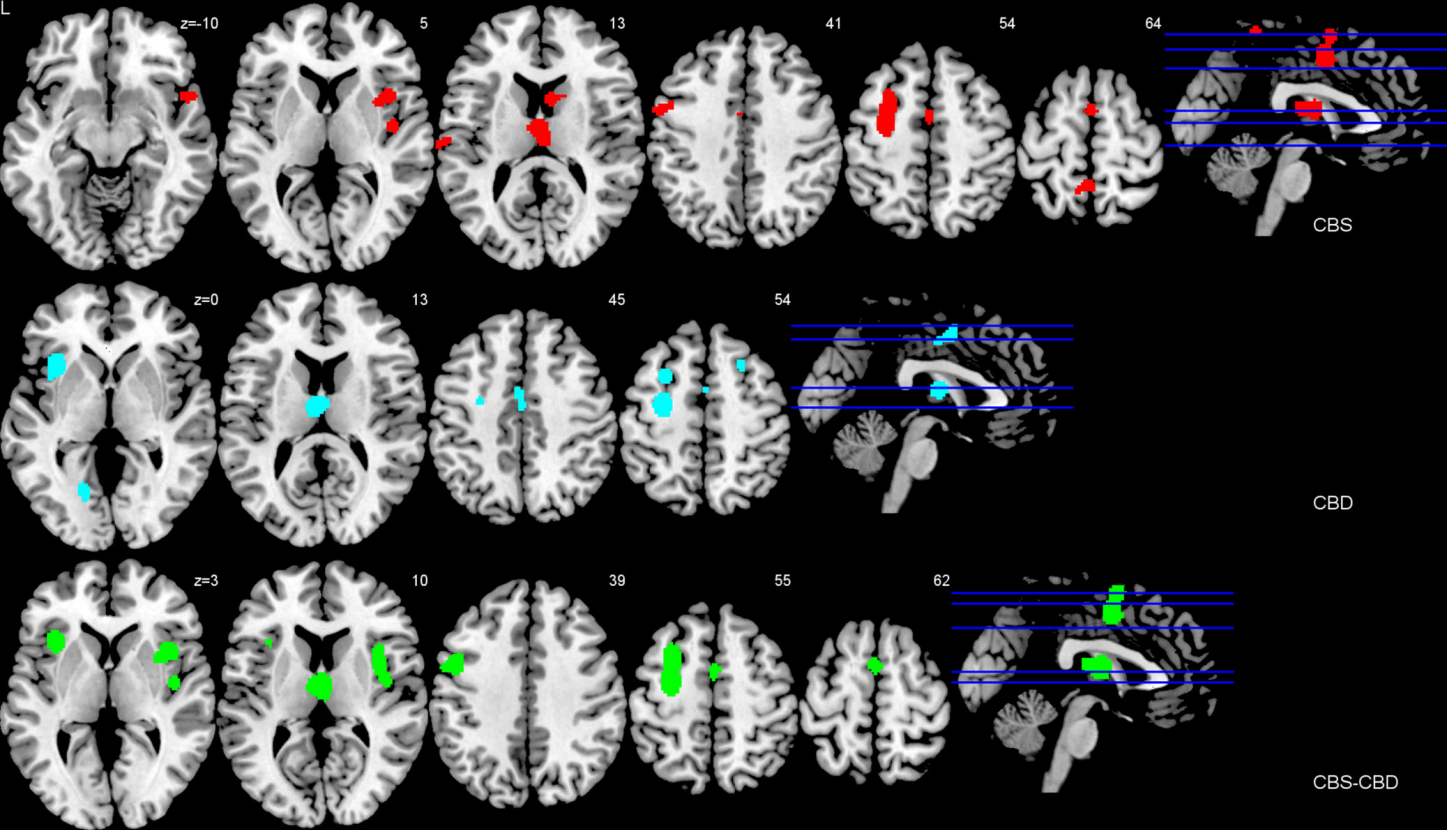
Impaired regions in CBS and CBD. Impaired brain regions in CBS and CBD in comparison with healthy control subjects–anatomical likelihood estimates meta-analyses. Atrophy was measured by MRI. The CBS analysis (*red*) included 184 patients from 17 cohorts contrasted to 265 healthy subjects. The CBD analysis (*blue*) included 34 patients from seven cohorts contrasted to 121 healthy subjects. The analysis of the combined cohort of CBS and CBD (*white*) included 200 patients contrasted to 318 healthy control subjects. Coordinates are reported in MNI space. *L* left
